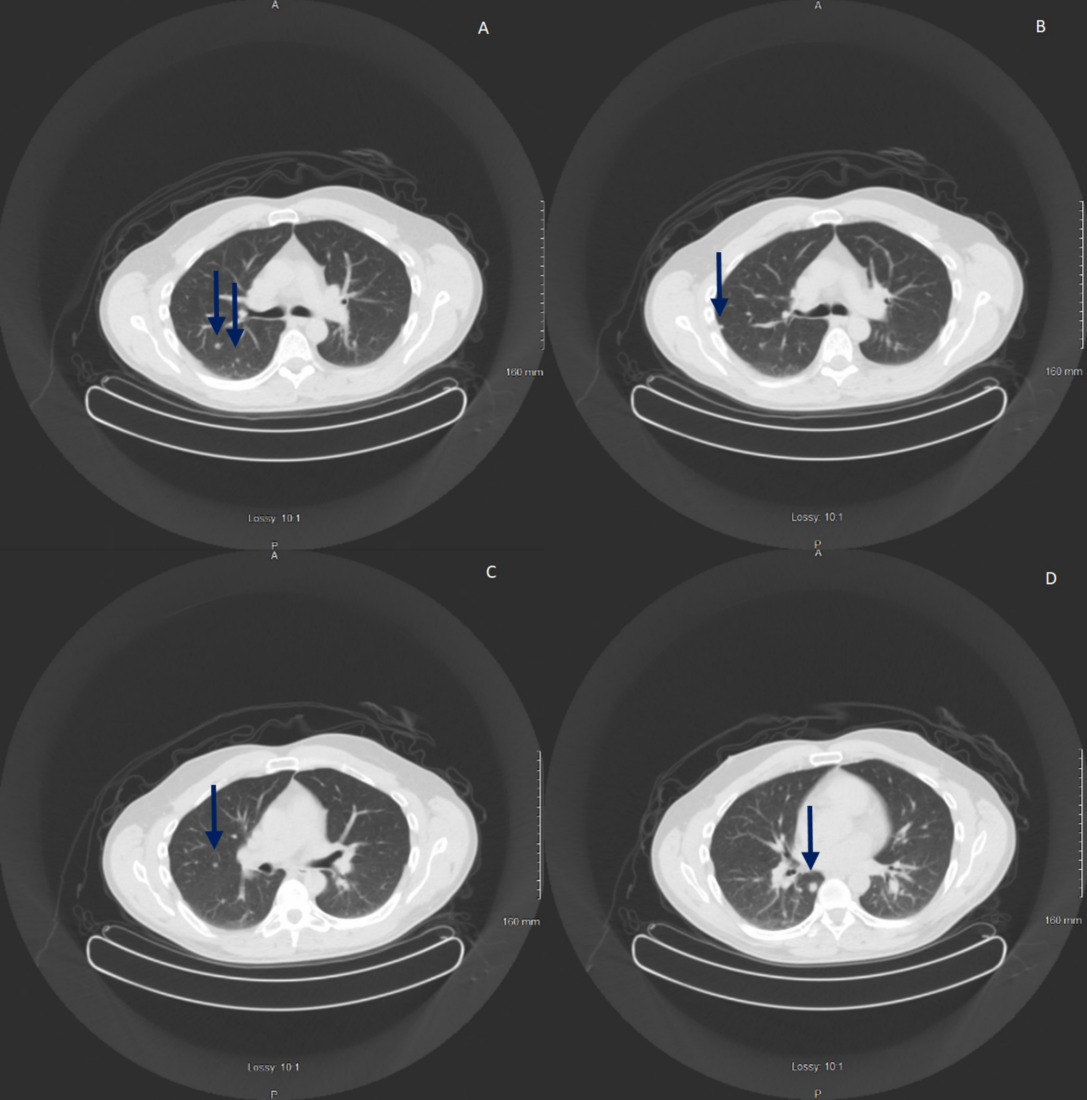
The figure shows the five lesions seen on the diagnostic CT scan consistent for metastatic colorectal cancer. A-D show the CT scan images and various locations.
